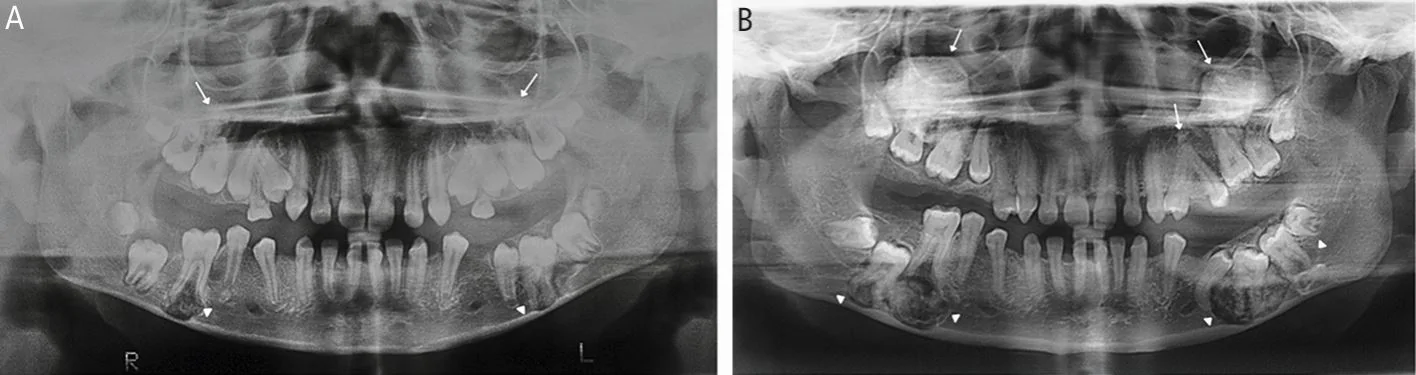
Panoramic radiograph of Case 1. (A) At 12 years of age, radiopaque lesions over the apical regions of the maxillary molars (arrows), and mixed radiopaque and radiolucent lesions between the root apices of the mandibular molars (arrowheads). (B) At 18 years of age, progression in size of the lesions associated with multiple unerupted teeth; the maxillary lesions appear completely radiopaque, with possible extension into the maxillary sinuses (arrows), whereas the mandibular lesions exhibit a mixed-density appearance (arrowheads)
